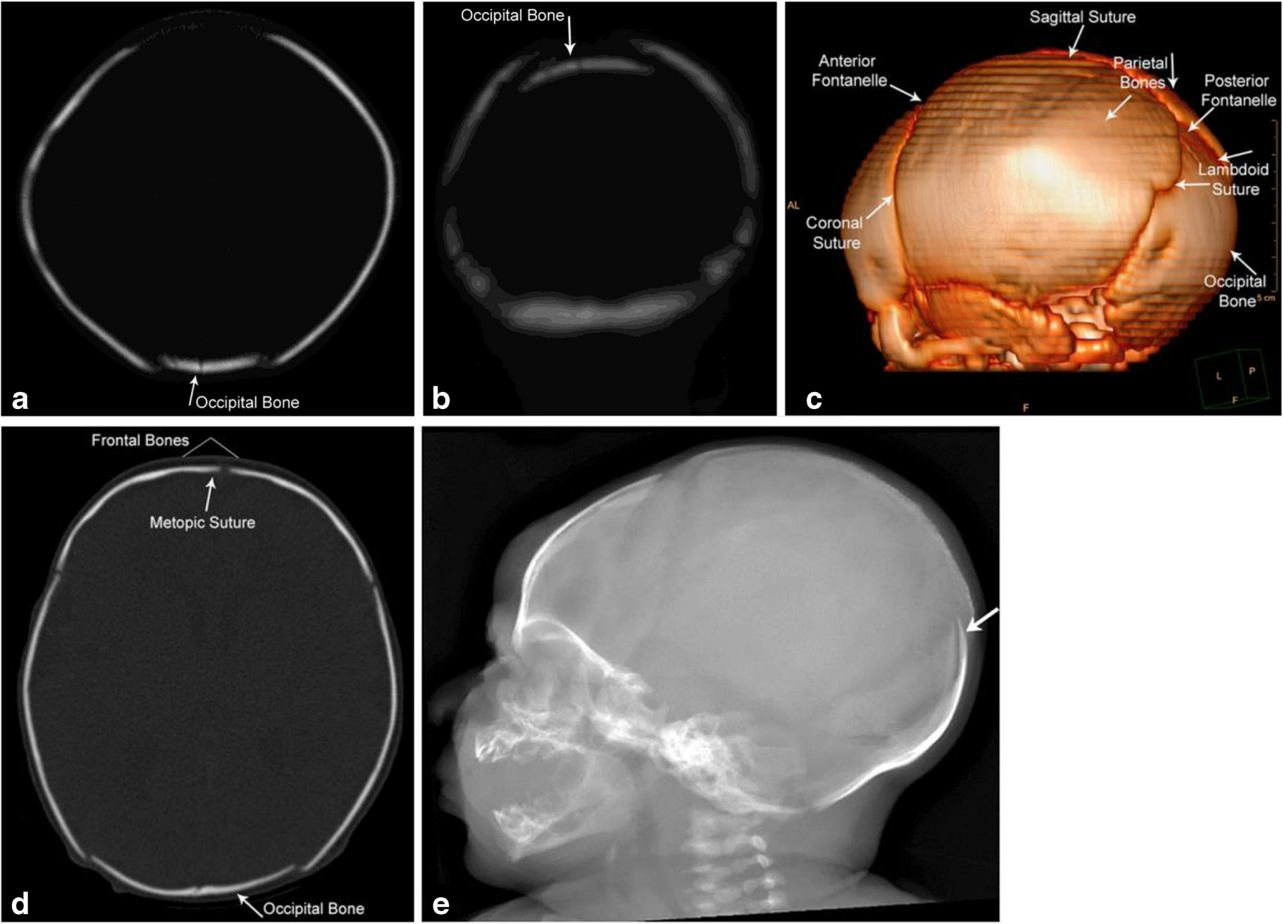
Molding of the skull post vaginal delivery Immediate post-delivery appearances of the skull on head CT. The occipital bone is slightly depressed with associated sutural overlap as seen on the axial and coronal CT images and the 3-D reconstructions (a-d). Lateral skull radiograph (e) demonstrates overlap of the occipital bone (white arrow)
